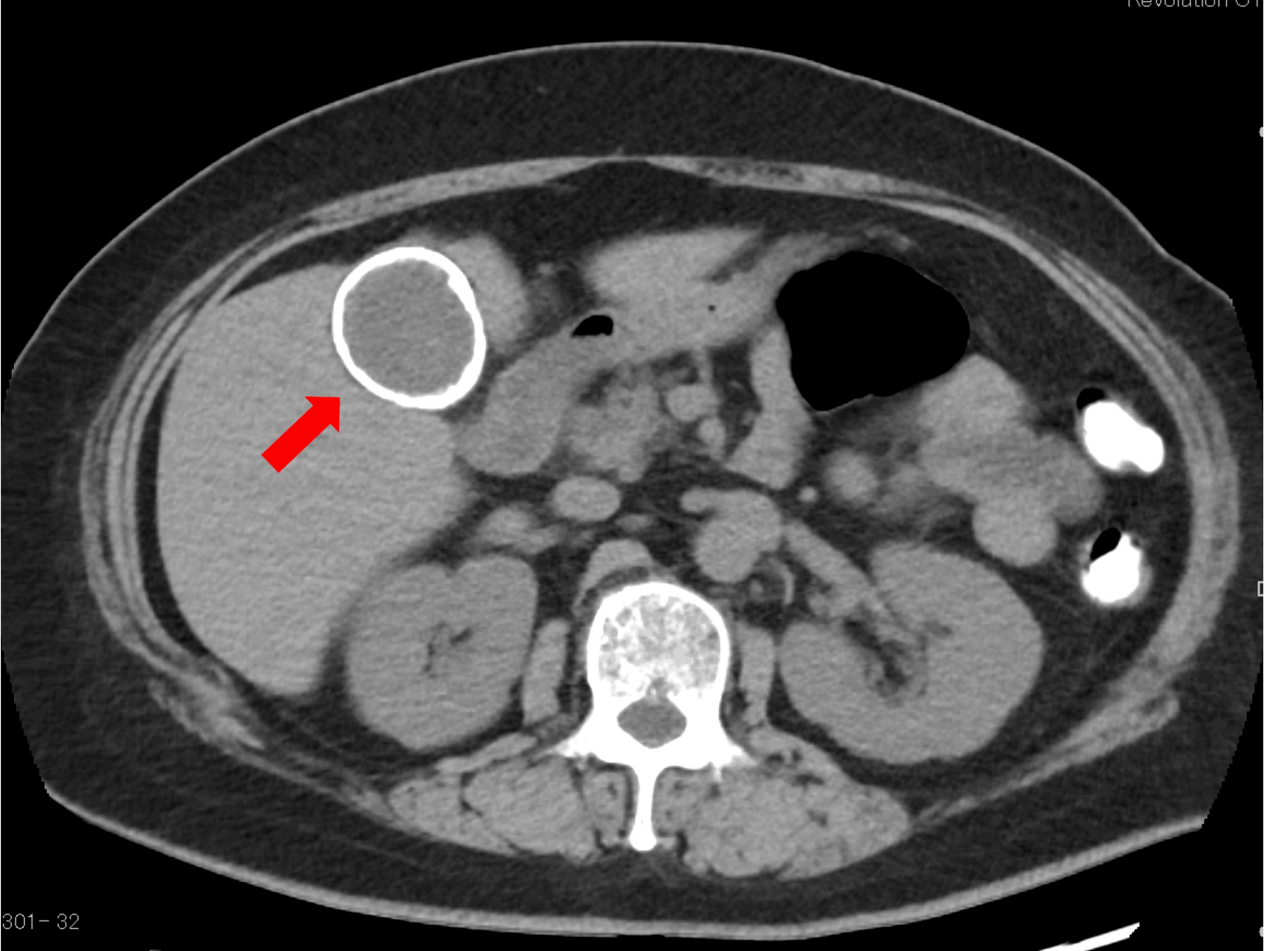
Axial view of the abdominal CT scan The axial view of the abdominal CT scan demonstrated calcification consistent with the gallbladder wall, forming a ringlike pattern (red arrow)
